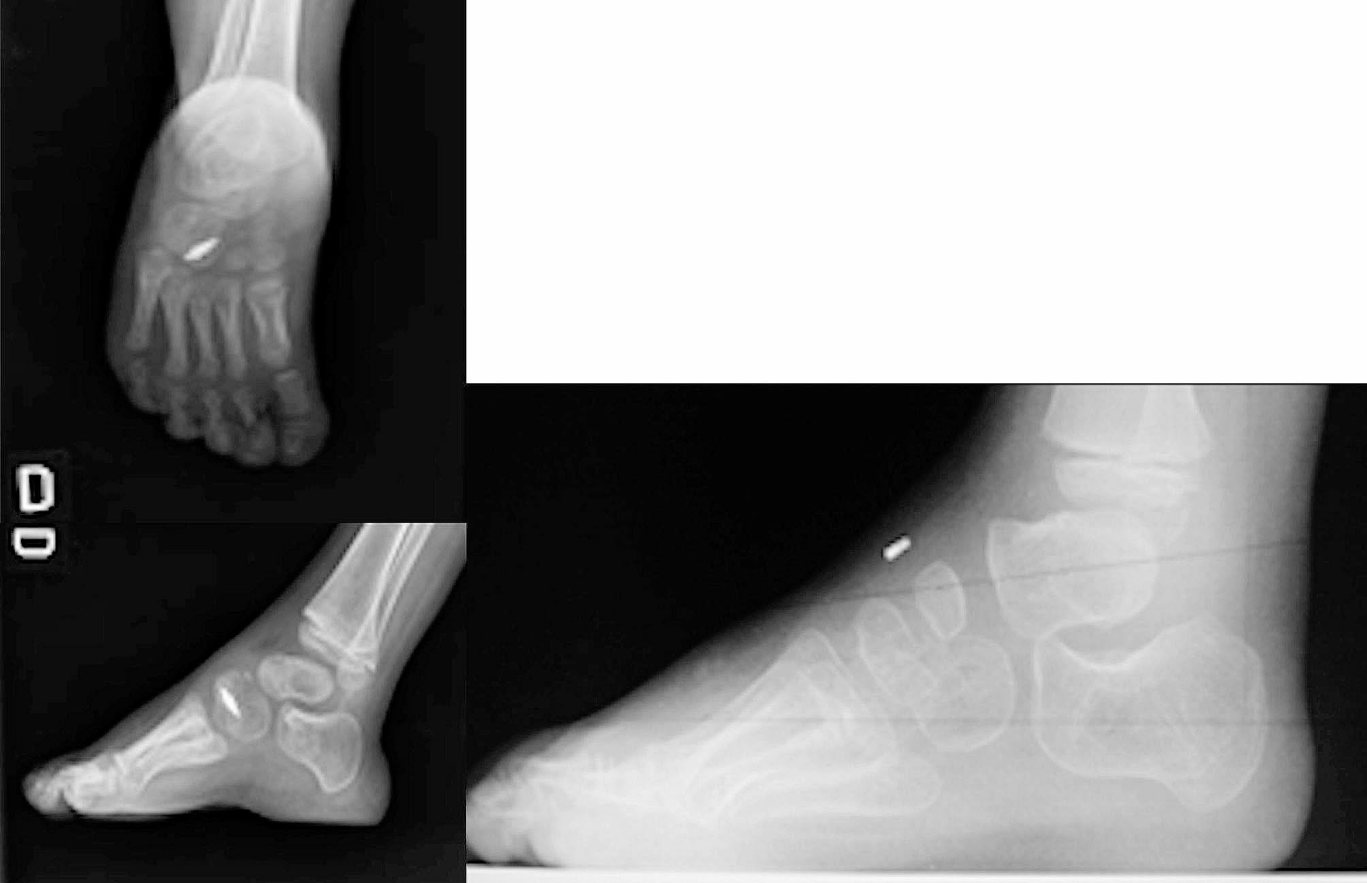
Immediate post-operative X-ray (left) and X-ray 4 months post-operative (right), demonstrating loosening of the bone anchor. Cortesy by Dr Cristina Alves from Portugal / Ponseti International Meeting Barcelona 2014
